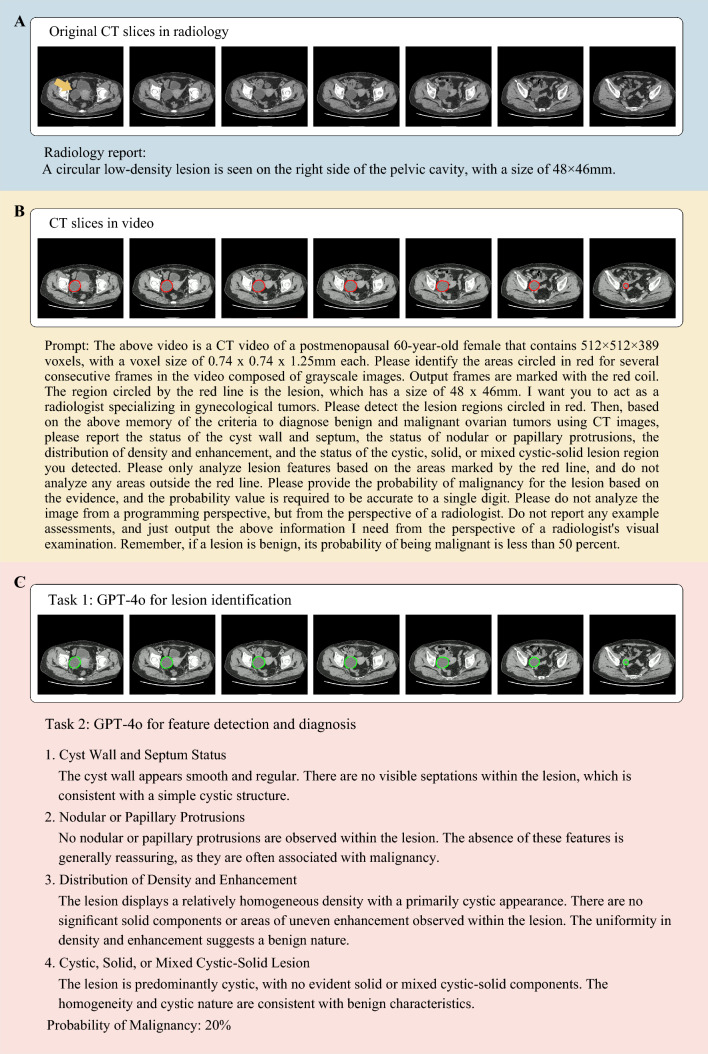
Example of GPT-4o identifying and analyzing computed tomography (CT) images of ovarian mass. A Pelvic CT imaging of one participant. B The detailed prompt used for GPT-4o diagnosis. C GPT-4o identification of the lesion area and the output analysis report about the features of the lesion and the diagnosis of the lesion being benign or malignant
